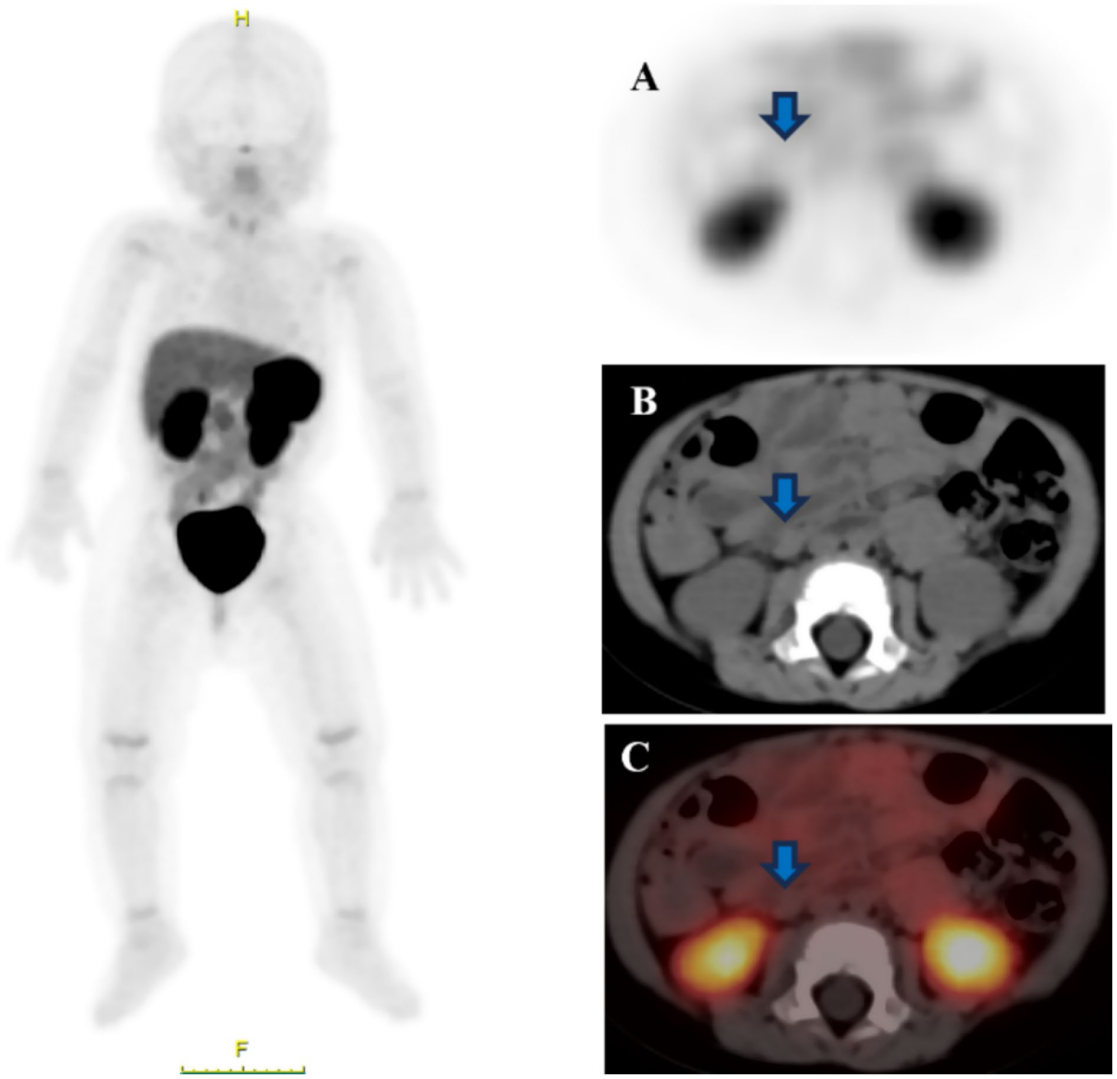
The primary site was located in the retroperitoneum in a child (female, 3 years old), and CT on the same machine showed [(B), blue arrow] a retroperitoneal lymph node with a diameter of approximately 0.5 cm; both PET and PET/CT [(A), blue arrow; (C), blue arrow] did not show any high uptake of nodal foci in the same area. The final reoperation pathology confirmed the diagnosis of metastatic neuroblastoma.
